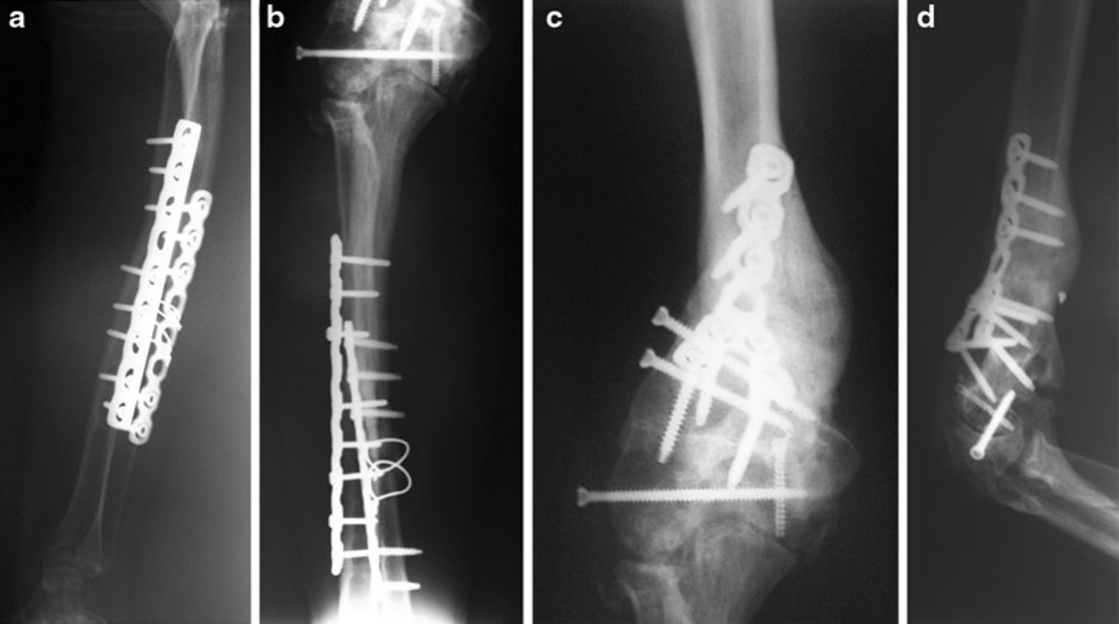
Anteroposterior and lateral radiographs showing healing of forearm (a, b) and humeral (c, d) fractures 1 year postoperatively
